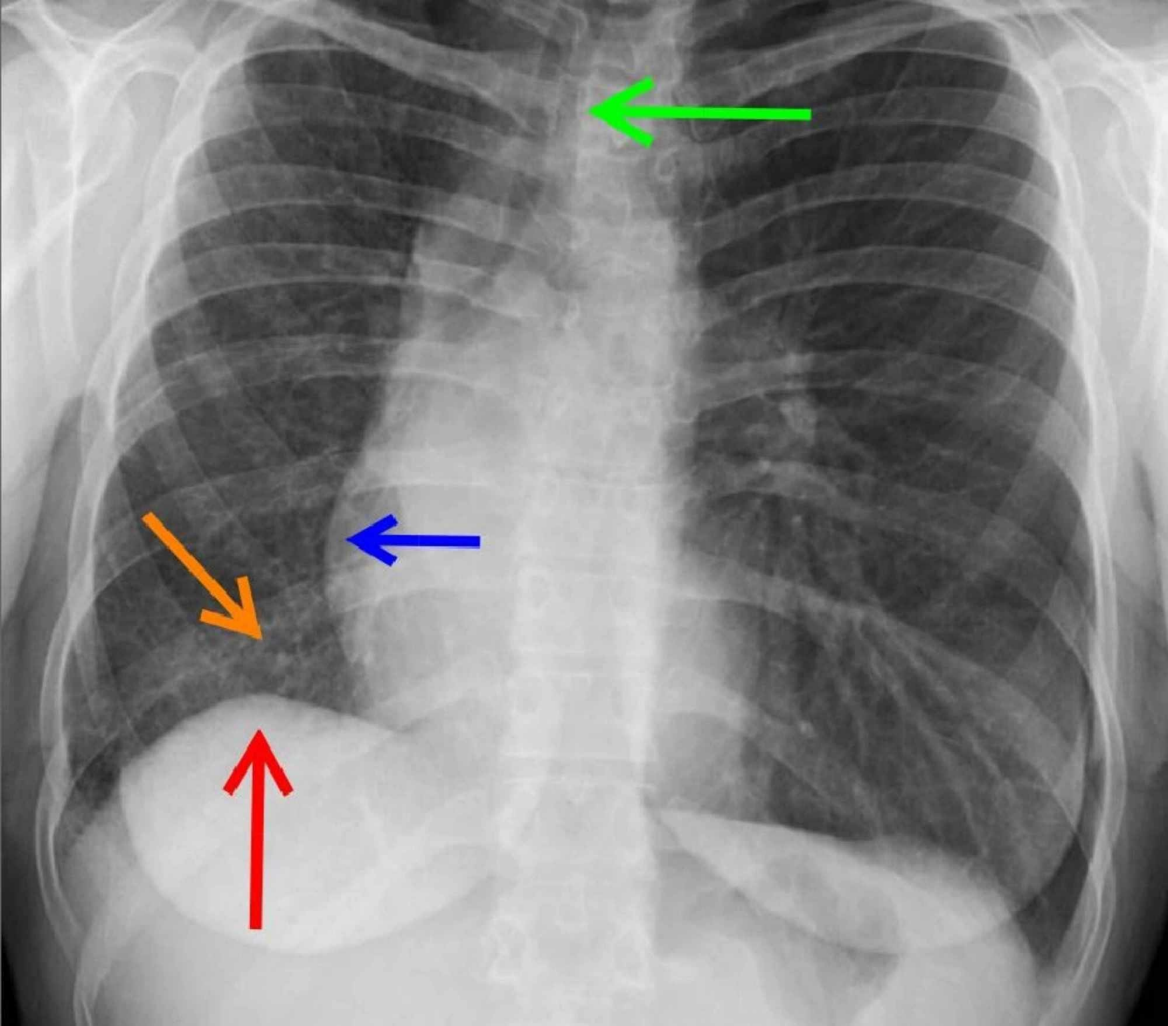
Chest X-ray evaluating causes for dyspnea. The radiograph shows right lung volume reduction manifested by tracheal deviation (green arrow), mediastinal shift (blue arrow), and elevation of the right hemidiaphragm (red arrow). There is also increased prominence of interstitial markings and reticulation of the right lung (orange arrow). The left lung shows no disease on plain film.
